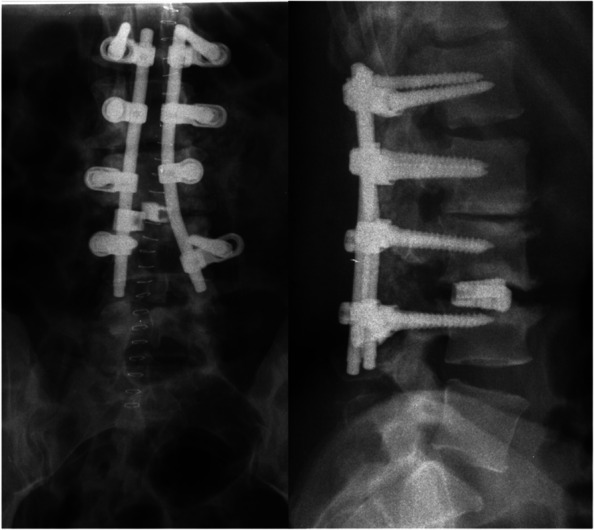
The postoperative x-rays showed a correct implant positioning after L1-L4 fusion with L3/4 PLIF (May 2007)
